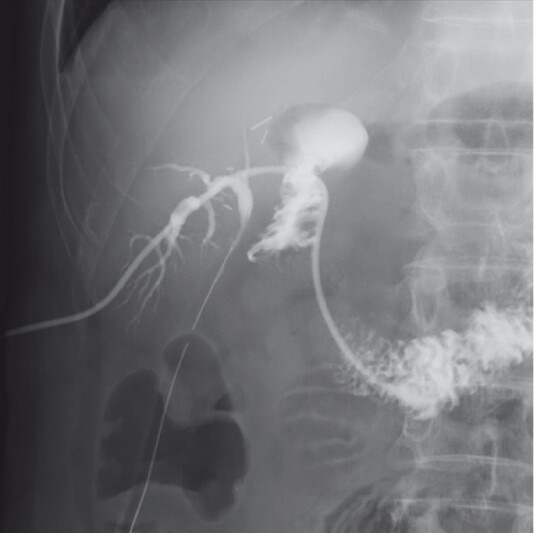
Duodenography via the percutaneous transhepatic biliary drainage (PTBD) route showing internal drainage successfully achieved via the rendezvous approach after insertion of a cholangioscope from the PTBD route allowed the guidewire coming from the endoscopic ultrasound-guided biliary drainage route to be grasped with biopsy forceps, so that a plastic stent could be deployed via the percutaneous route into the duodenum along the guidewire.
